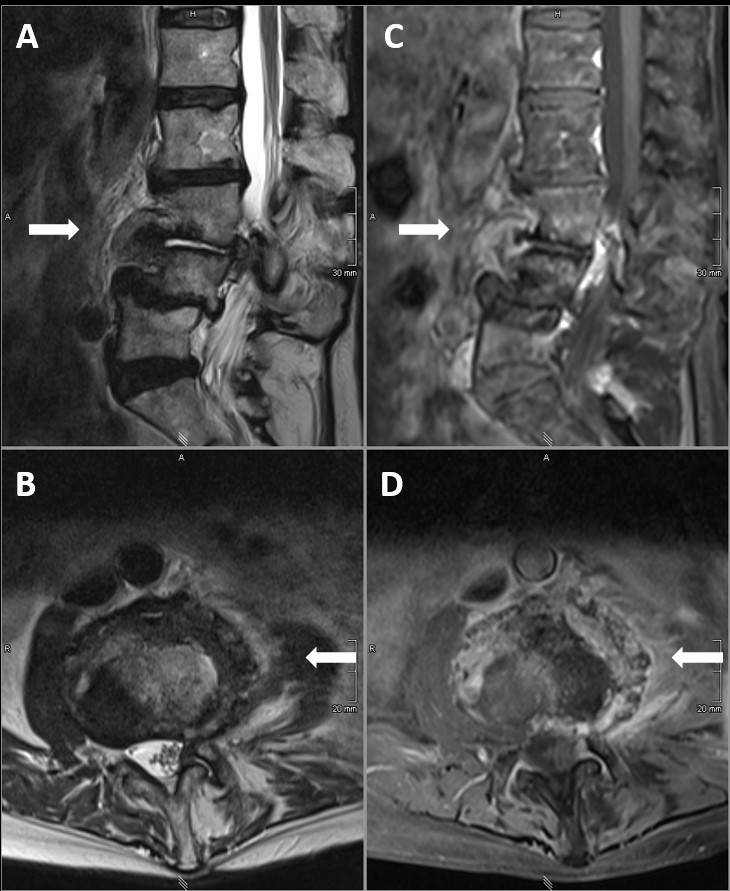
MRI (T2w images sagittal (a) and axial (b); T1w
